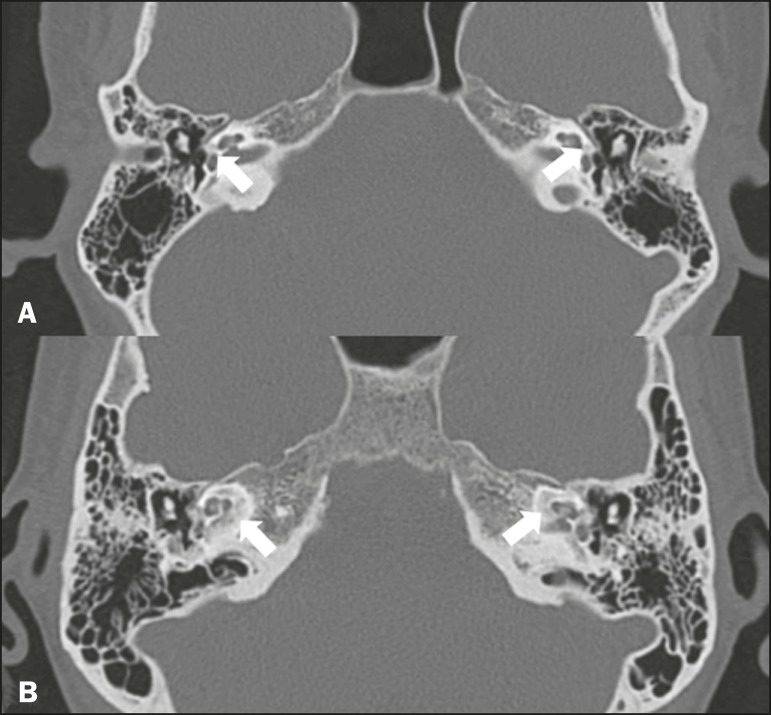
Otosclerosis. A: Axial CT scan, with bone window
